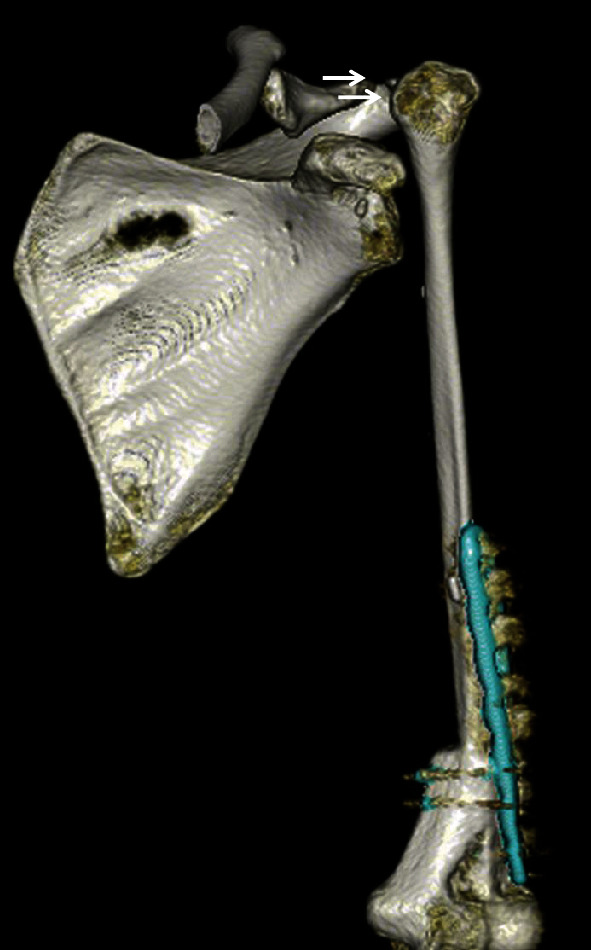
Postoperative 3D CT scan 3D CT scan showing the clavicle, scapula, humerus, and grafted fibula. Arrows indicates the location of the sling loop.
